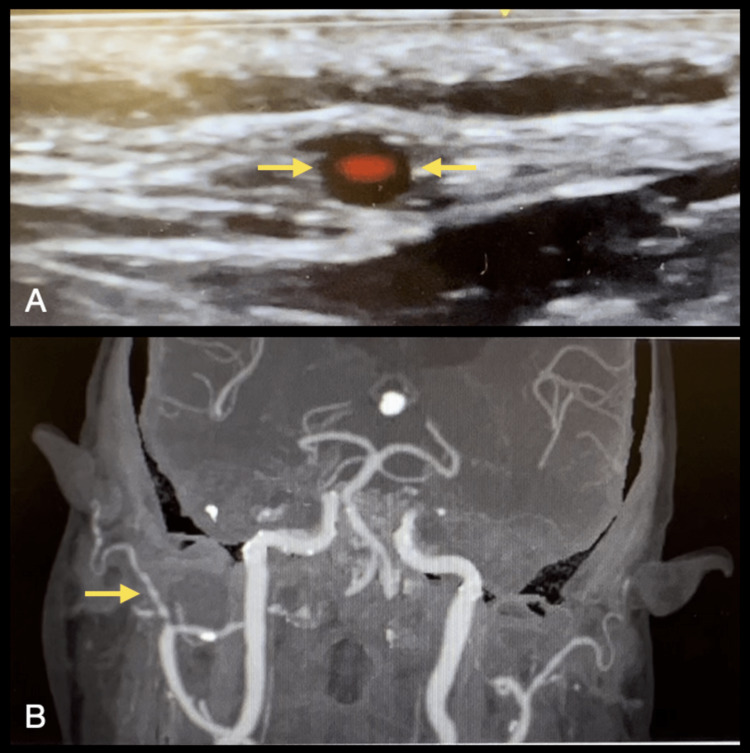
(A) Halo sign of the superficial temporal artery in an 89-year-old man with a history of polymyalgia rheumatica who presented to the ED with an acute painless right monocular vision loss and temporal tenderness. (B) Contrasted CTA revealed a beaded appearance of the right superficial temporal artery suggesting GCA. Image credit: Authors; consent taken from the patient with confirmed GCA. CTA: computed tomography angiography; GCA: giant cell arteritis
